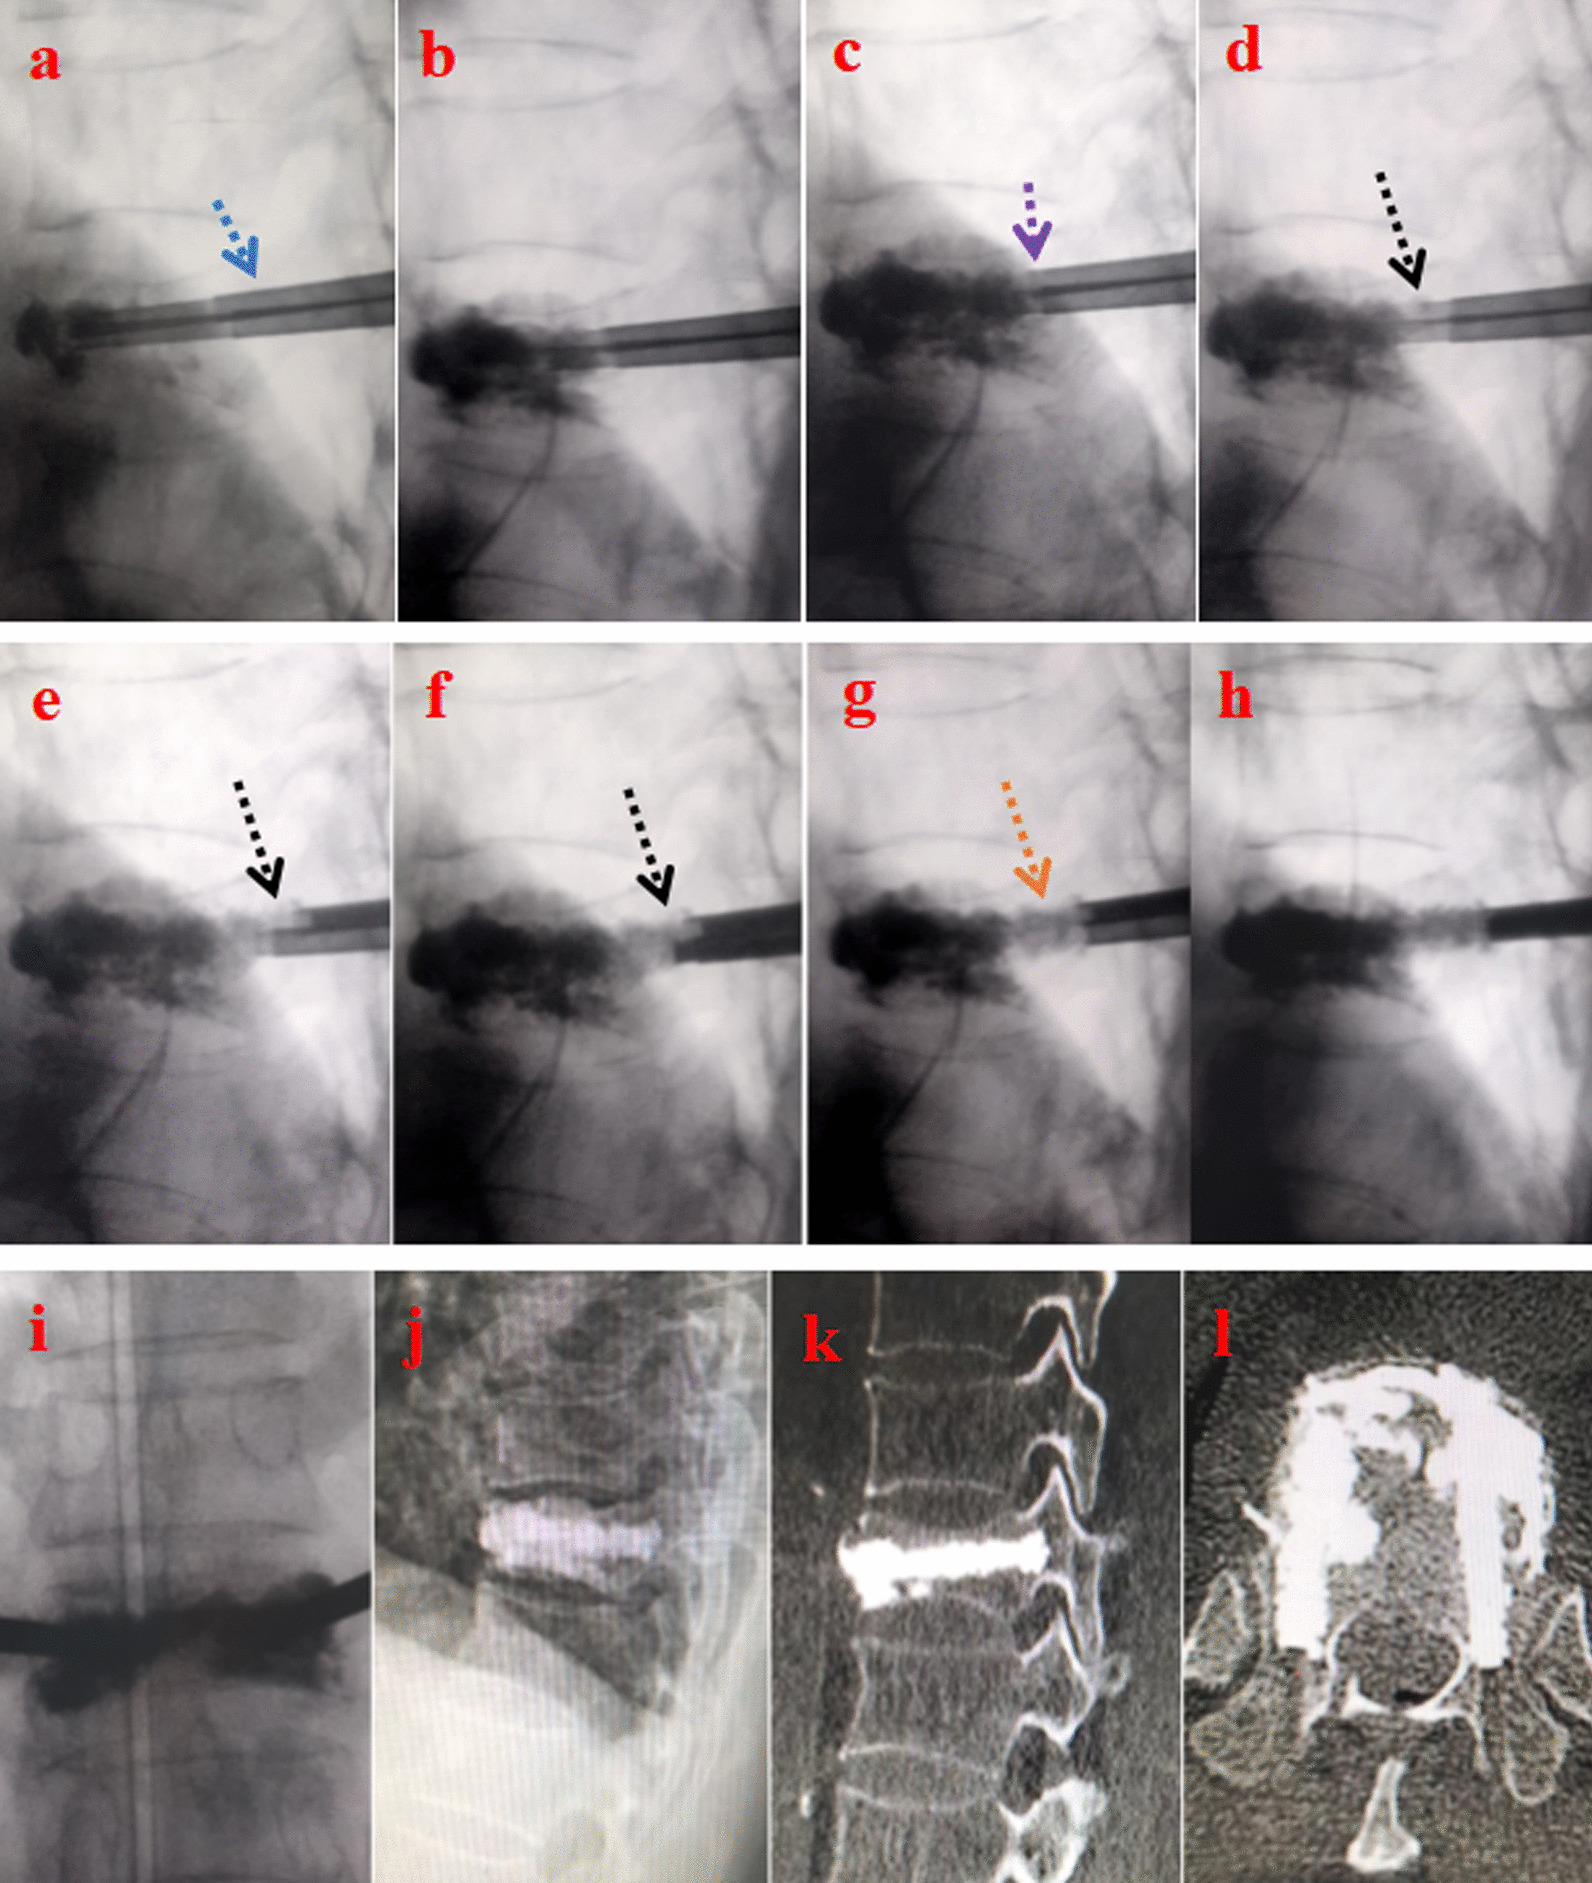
Special operation of the PKCPP surgery, postoperative lateral radiographs, and CT scans. a Making the two working cannulas un-overlapped by slightly adjusting C-arm’s position (blue arrow); b injecting the bone cement of the anterior two-thirds of vertebral body; c–g the procedure of pediculoplasty shows that the cement distribution is columnar shape; h–i intraoperative standard anteroposterior and lateral radiographs show the augmented vertebral body. j–l Postoperative lateral radiographs and CT scans show that the distribution of bone cement is like two pedicle screws bridging the cement-augmented vertebral body
